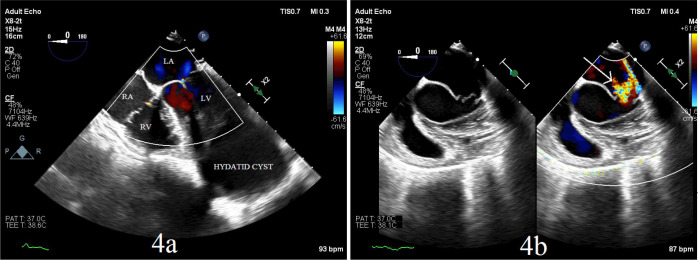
a) Transesophageal echocardiography image showing large left ventricular
